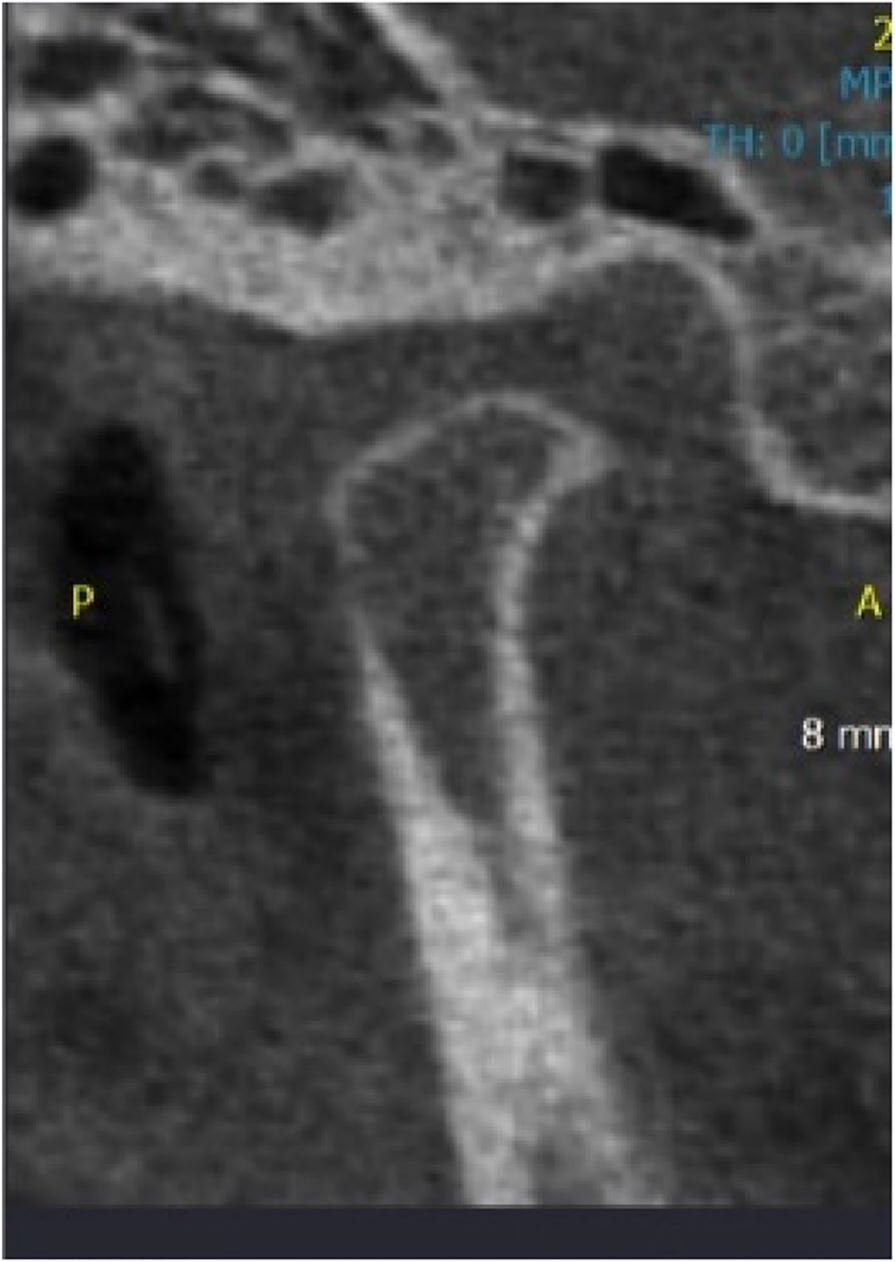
Sagittal CBCT view of right condyle showing present of osteophyte in anterior border of condyle
